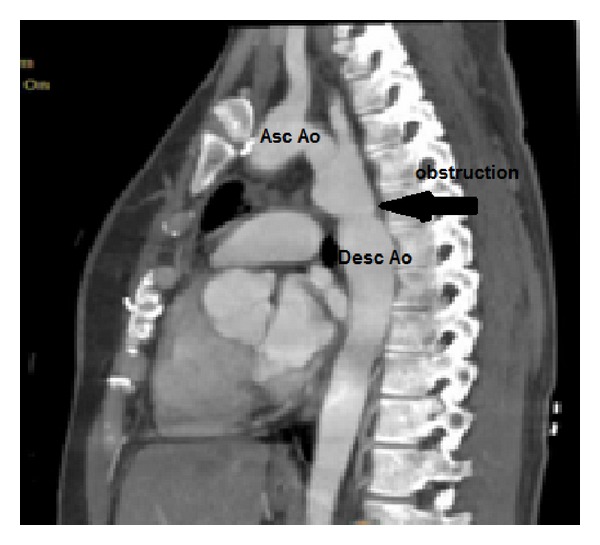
Computed tomography angiography demonstrates the postductal coarctation of the aorta. The descending aortic arch is tortuous and the diminutive left subclavian artery arises proximal to the coarctation.
